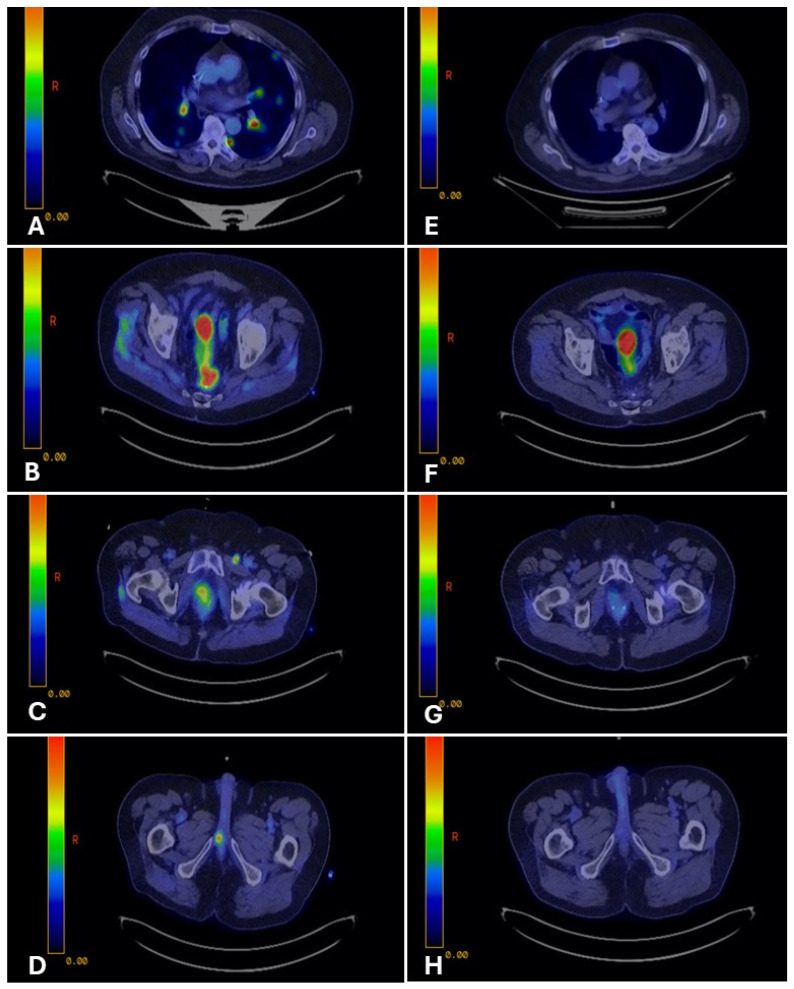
PET-CT imaging before and after fluoropyrimidine-based therapy in Case 1. Legend: Representative PET-CT images demonstrate FDG-avid disease at multiple sites before therapy and the response after fluoropyrimidine-based treatment. Pre-treatment (Panels (A–D)): FDG-avid disease involving bilateral lungs (A), presacral pelvic mass (B), pelvic lymph nodes (C), and right penile crus (D). Post-treatment (Panels (E–H)): Restaging PET-CT images show complete metabolic response at all previously involved sites, with resolution of FDG uptake (E–H).
